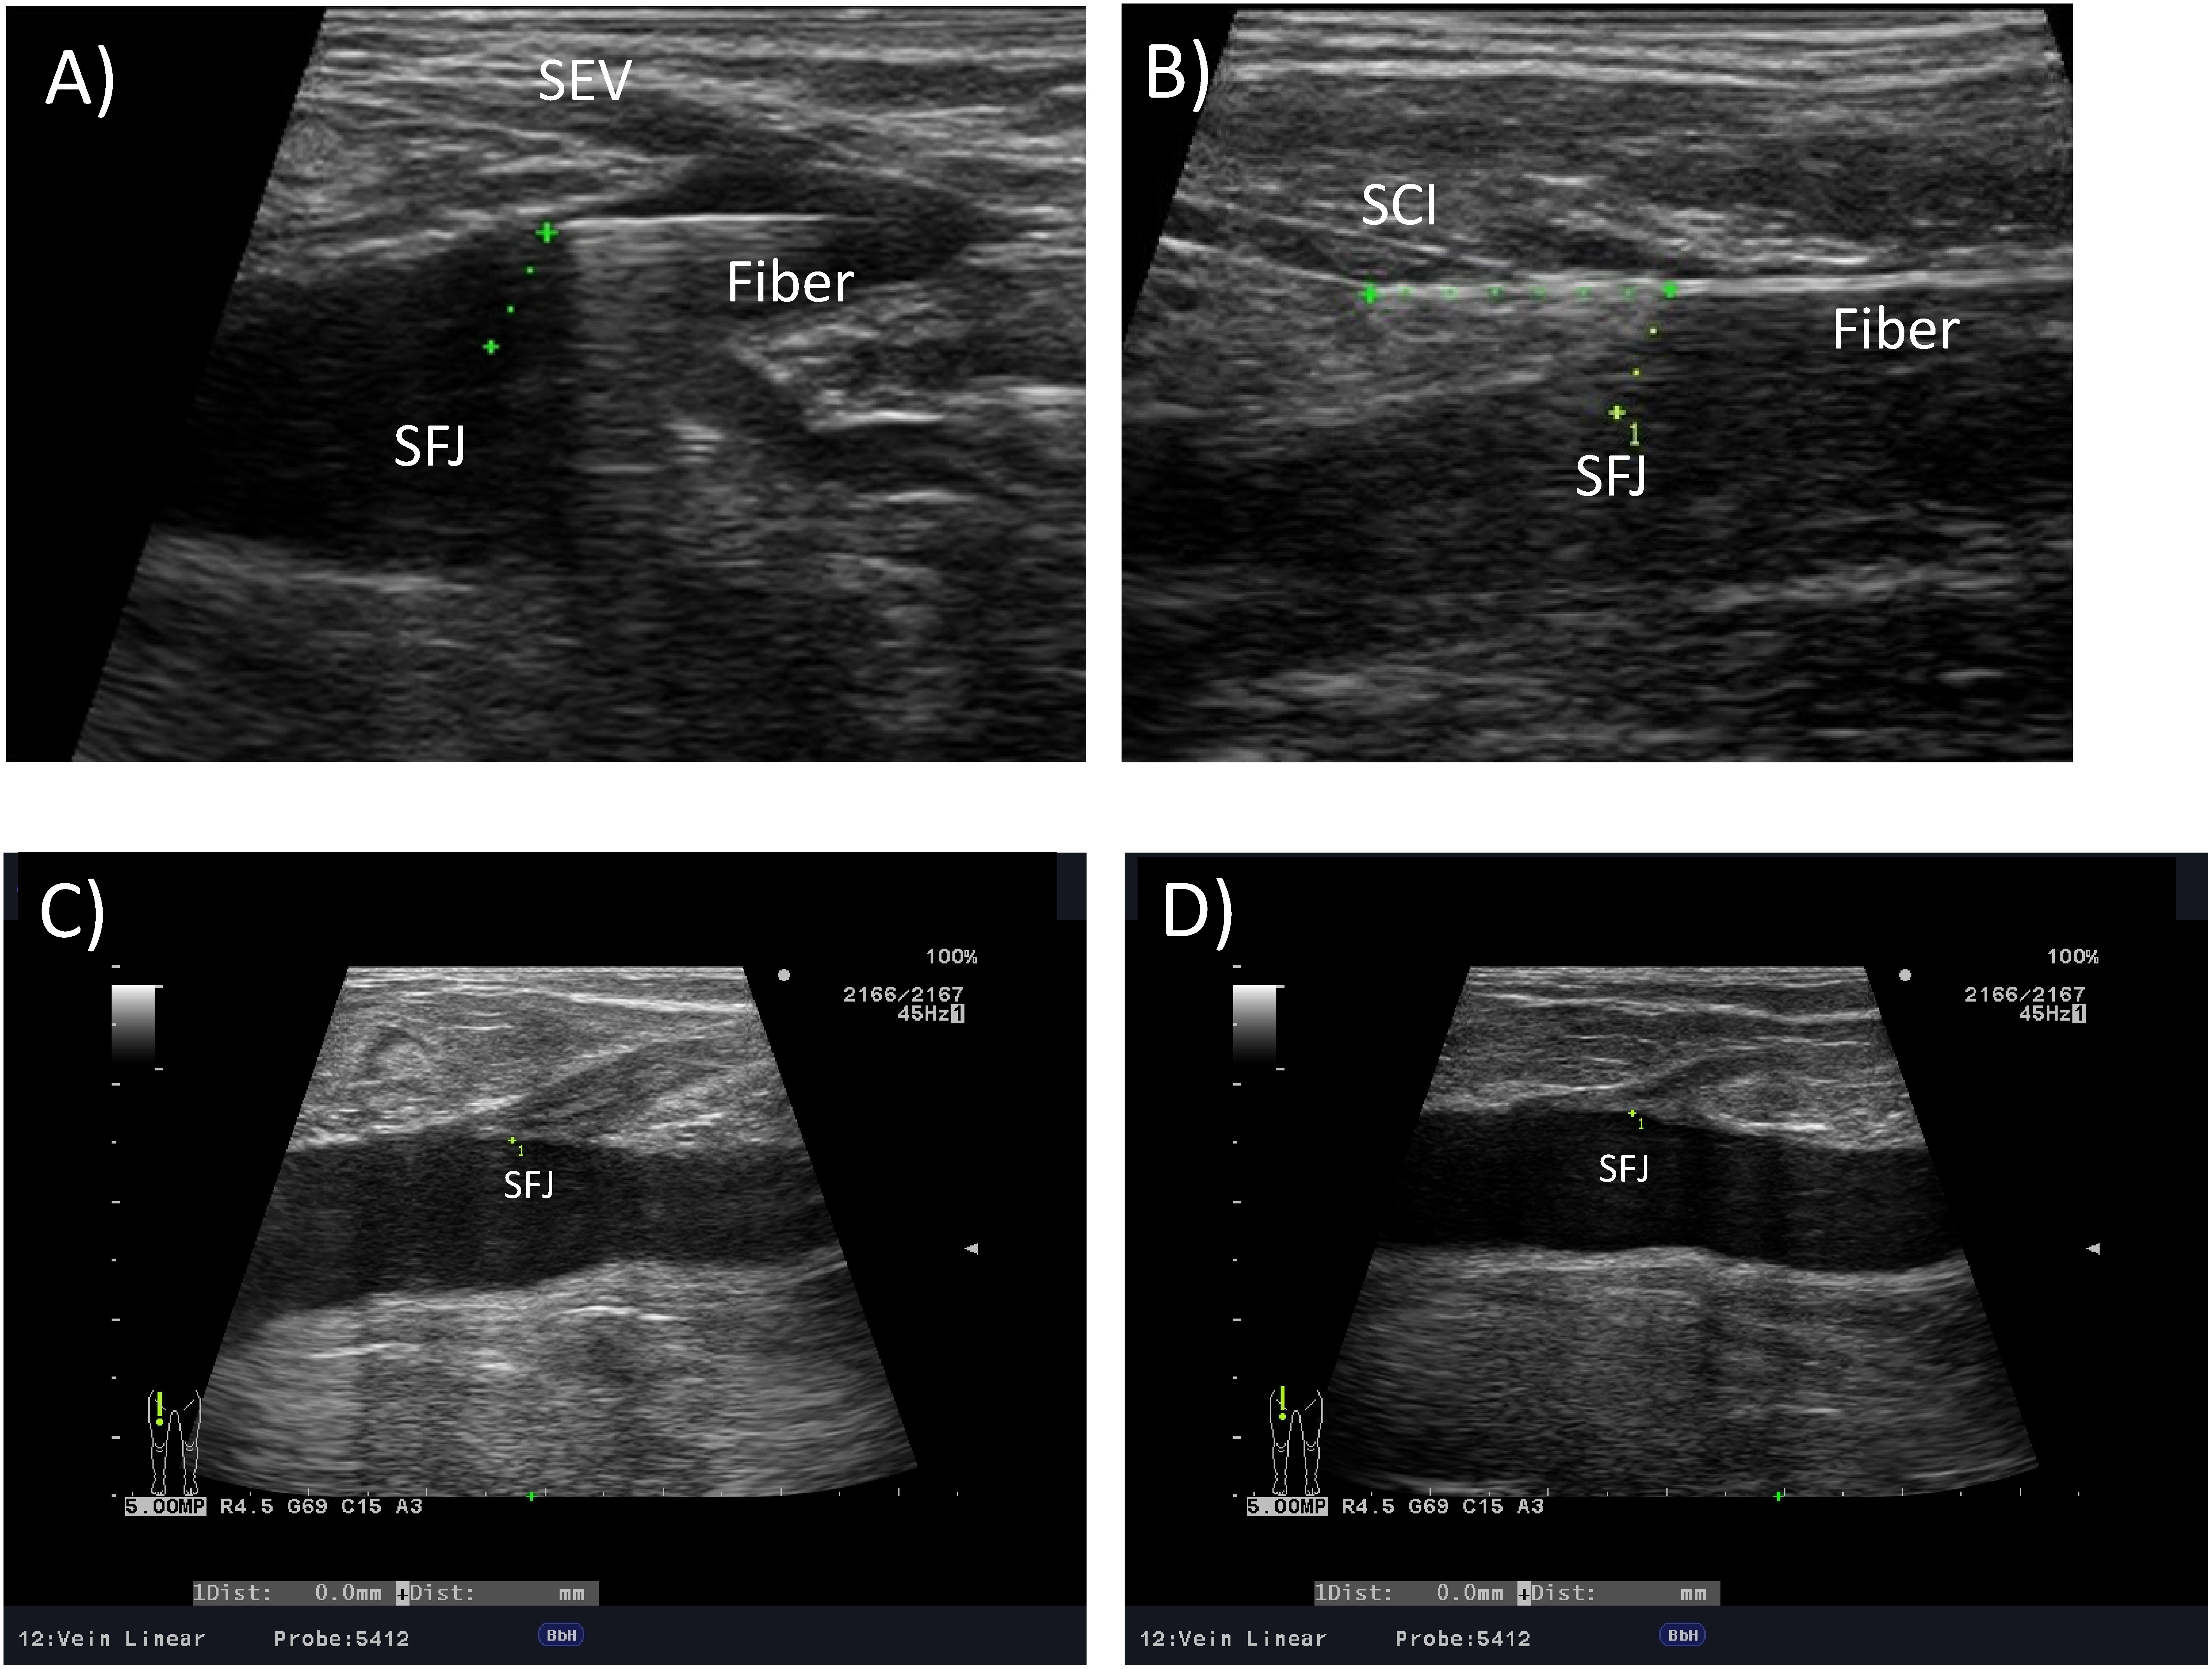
Fig. 1 Positioning of the fiber tip during endovenous thermal ablation and flush occlusion. (A) Duplex ultrasound shows the fiber tip positioned close to the saphenofemoral junction (SFJ) proximal to the superficial epigastric vein (SEV) for laser crossectomy. (B) Duplex ultrasound shows the fiber tip inserted into the superficial circumflex iliac vein (SCI) for cranial tributary ablation. (C) Duplex ultrasound shows flush occlusion of the great saphenous vein obstructed just at the SFJ 3 months after laser crossectomy with concomitant superficial circumflex iliac vein ablation. (D) The great saphenous vein remained occluded at the SFJ 6 months after endovenous ablation (C and D are images from the same patient).
